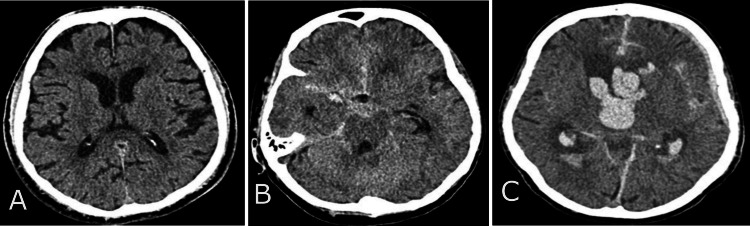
Intraventricular blood or traumatic subarachnoidal haemorrhage (SAH) CT findings Intraventricular and subarachnoidal space normal findings (A); subarachnoidal hemorrhage (SAH) (B); intraventricular hemorrhage (IVH) and SAH (C).
